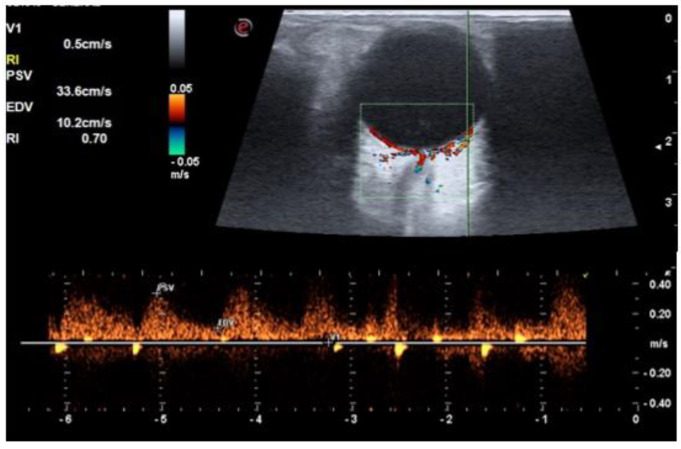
A color Doppler image ultrasound of a diabetic patient without retinopathy showing resistive index of 0.70 in short posterior ciliary arteries.
